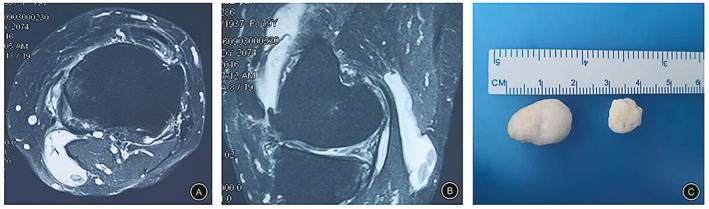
A 63‐year‐old female who presented with distension of the right knee 9 months after resection of a hemangioma in the popliteal fossa of the right lower limb. The patient was treated by knee arthroscopy. (A & B) Preoperative MRI showing a popliteal cyst with loose bodies formation on the right knee. (C) The internal loose bodies were removed when the cyst was treated under arthroscopy, suggesting that the cyst communicated with the articular cavity through the valve opening.
